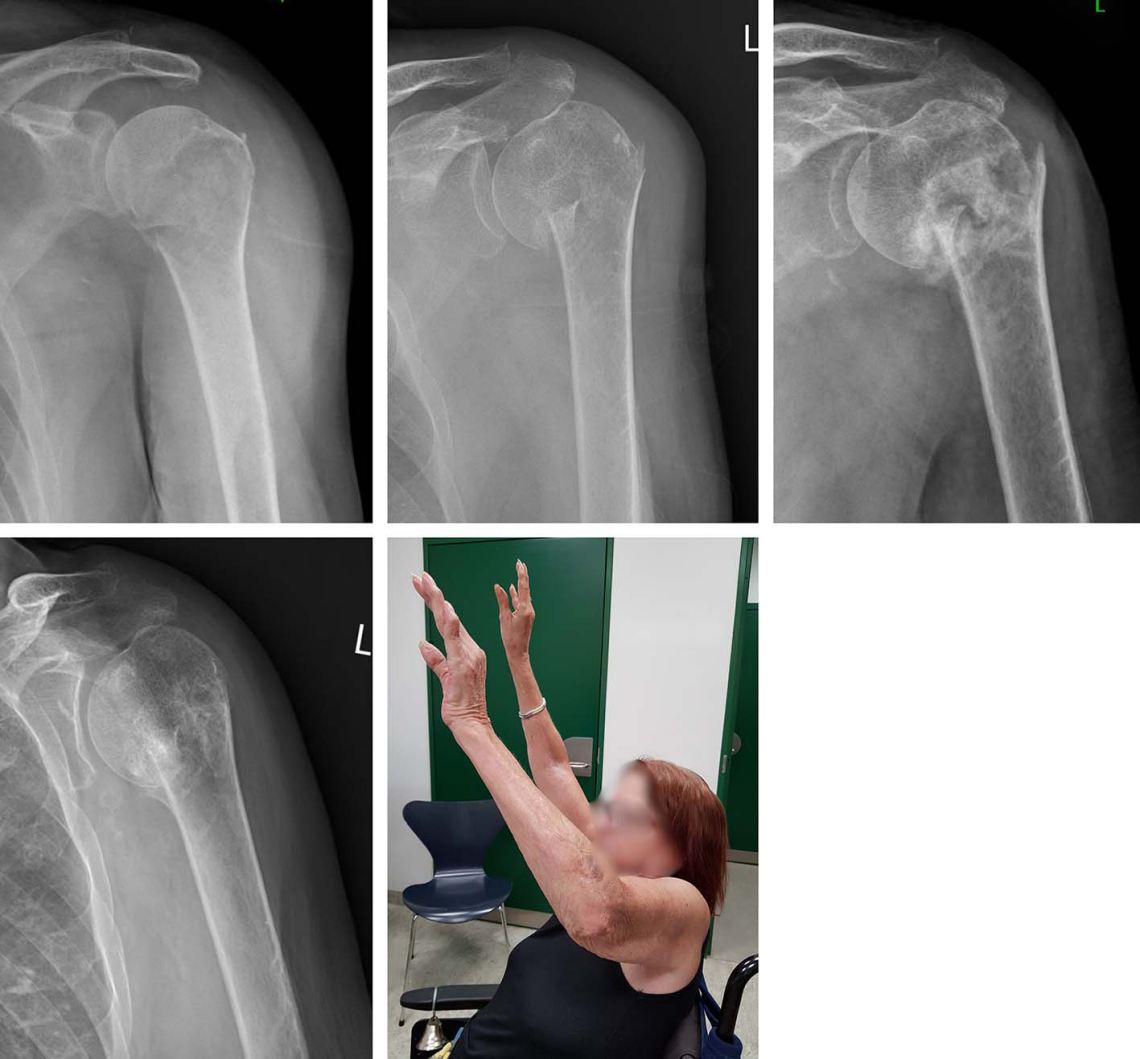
A progressive varus collapse of a minimally displaced fracture in a disabled nursing home resident. Despite the collapse and partial resorption of the humeral head, the pre-injury function was restored. Radiographs at admission, 2 weeks, 6 weeks, and 24 weeks. Clinical photo at 24 weeks.
